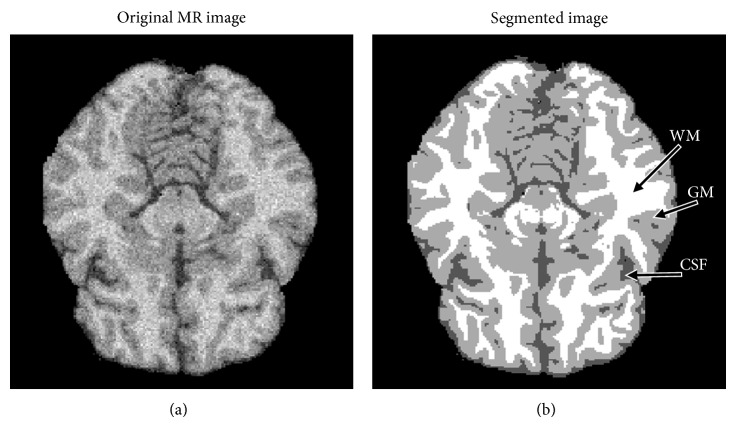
An example of the brain MRI segmentation with an original MR image (a) and segmented image with three labels: WM, GM, and CSF (b).
